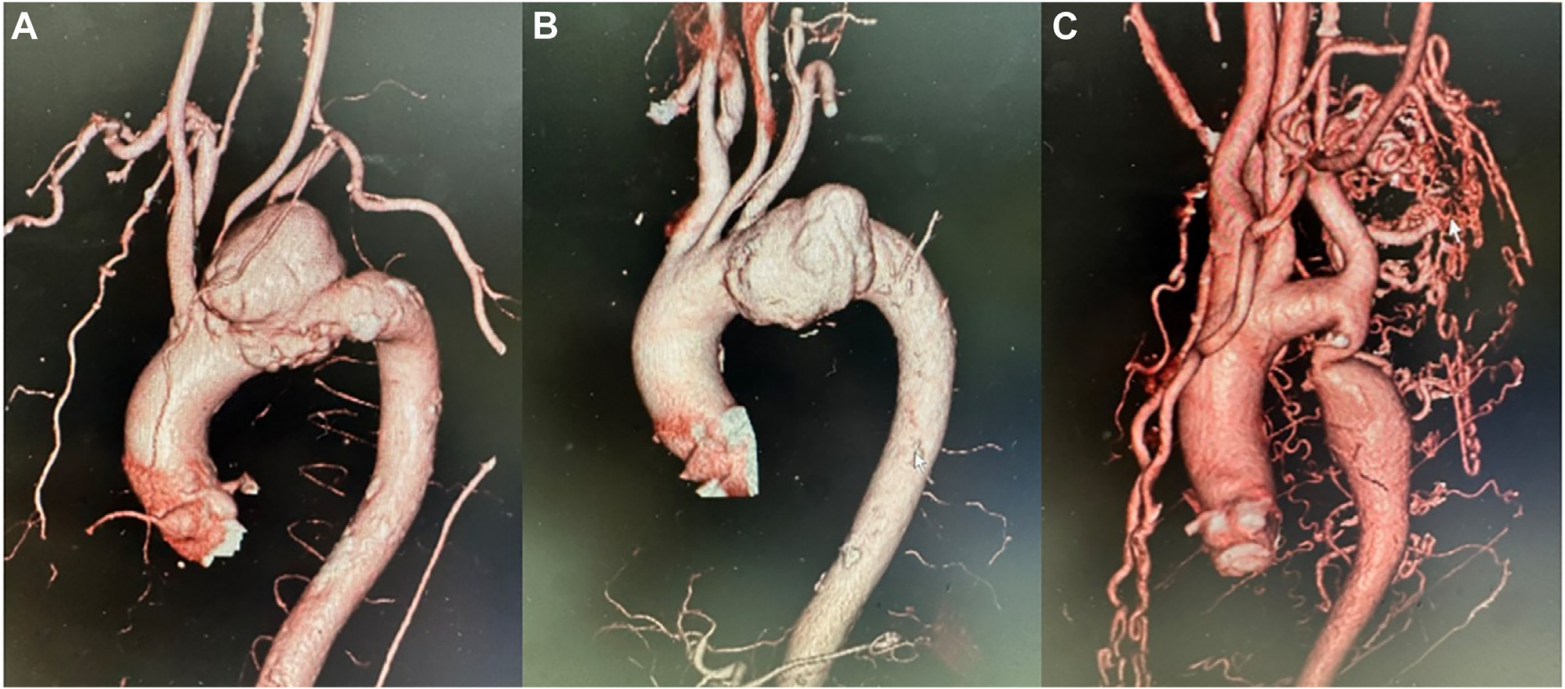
Preoperative computed tomography image of each patient. (A, B) Patients 1 and 2 with infectious aortic aneurysms. The suspected infectious aortic aneurysms are located at the distal arch. (C) Patient 3 with aortic coarctation.
